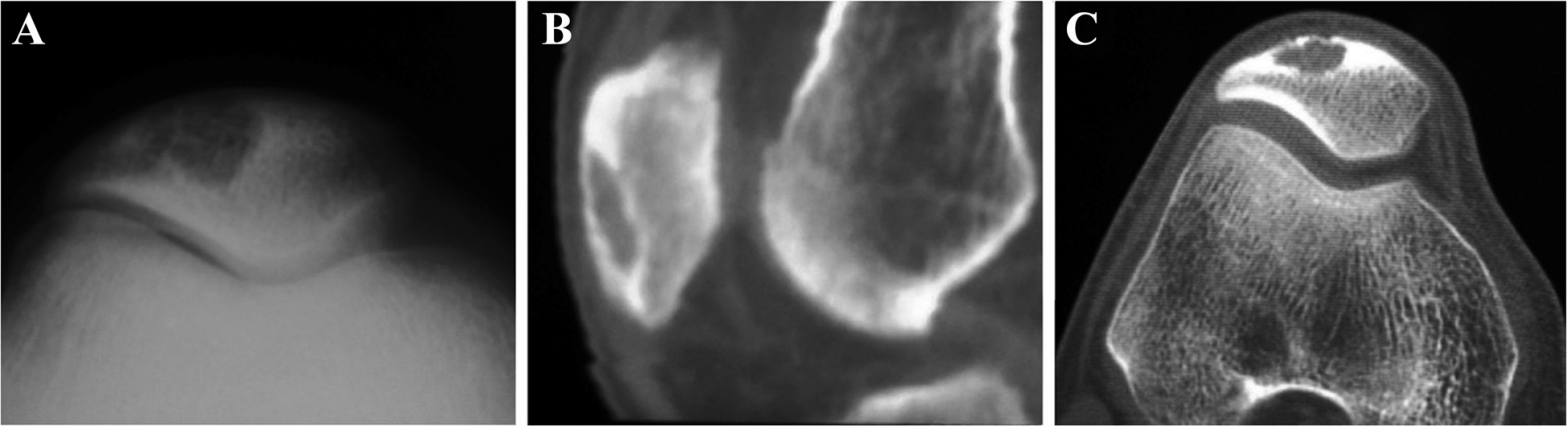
Patellar hemangioma. (A) Radiographs show a lytic lesion with sharp margins, thinning cortex, and sclerotic rim. (B, C) CT results accord with the radiographic results.
